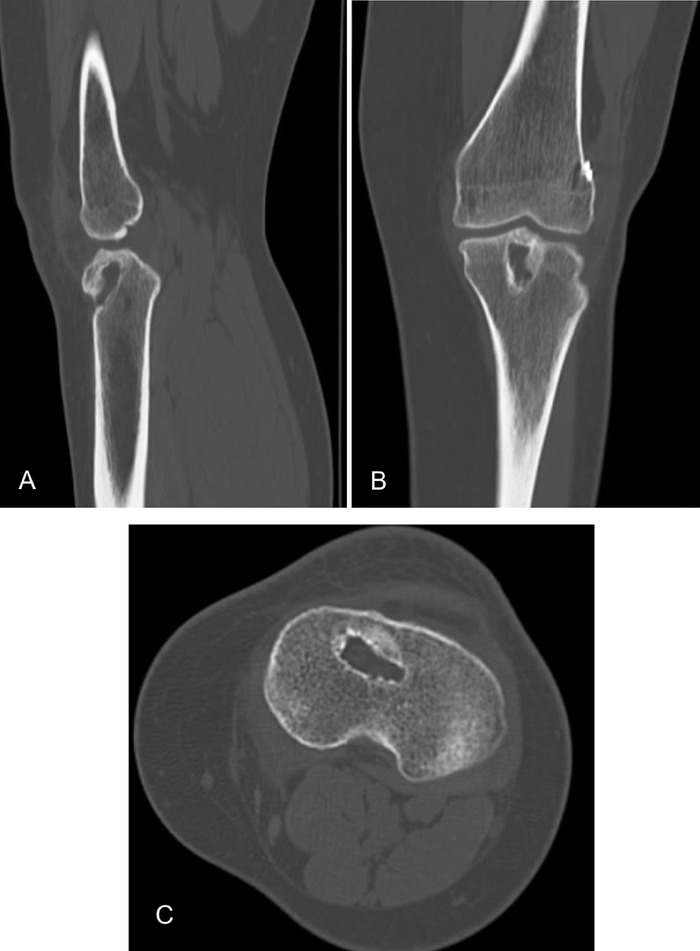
Computed tomography scans of the left knee show tibia tunnel in (A) sagittal, (B) coronal, and (C) axial views.
